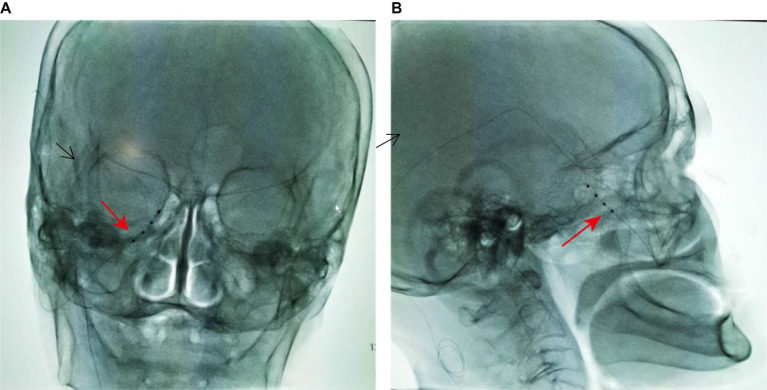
Radiographs of the maxillary branch electrode implanted through the foramen rotundum region (arrow). (A) Skull anteroposterior, C-arm angulation (LAO 10°, CRAN 6°). (B) Skull lateral, C-arm angulation (LAO 106°, CRAN 3°).
